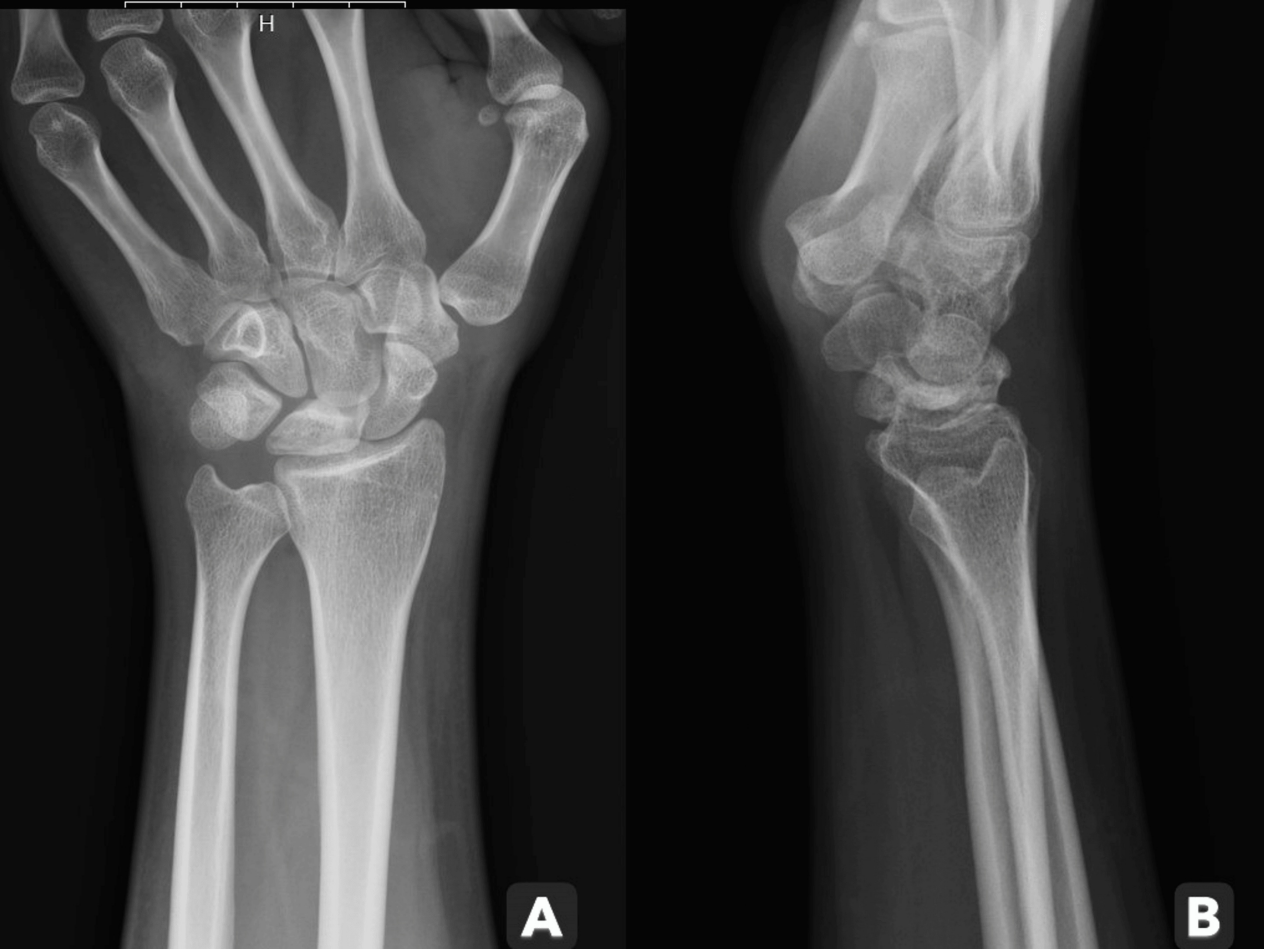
Initial left wrist radiograph indicating lunate mild sclerosis. (A) Anteroposterior view. (B) Lateral view.
